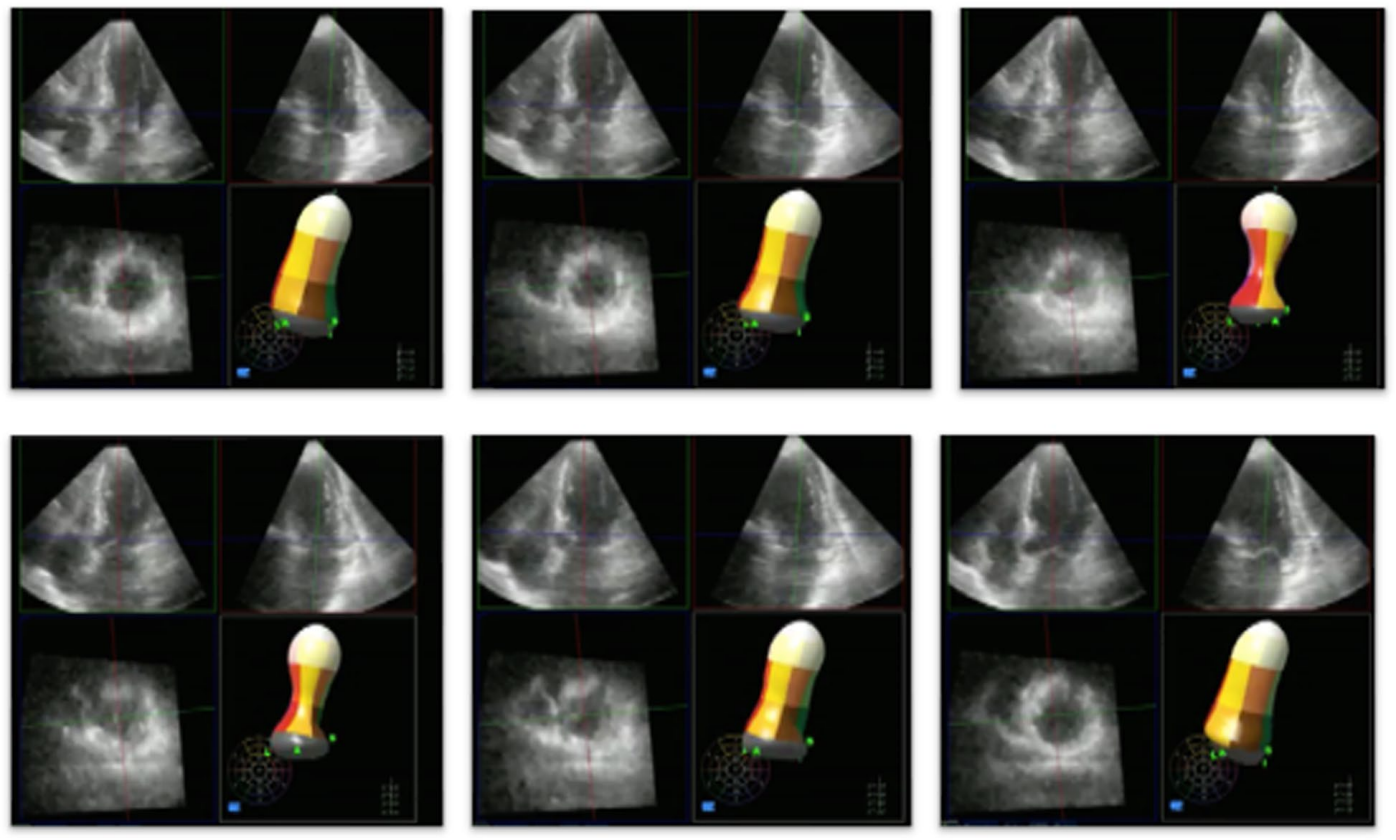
Subsequential echocardiographic 3D images of ejection fraction of a typical form of TTS
